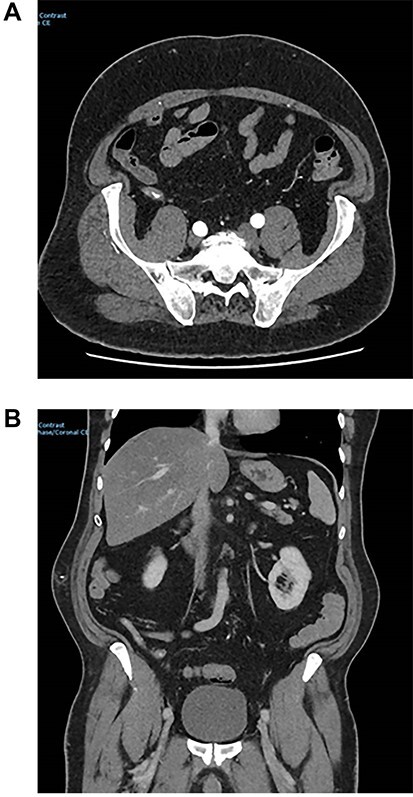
CT images of arterial appendix blush.
